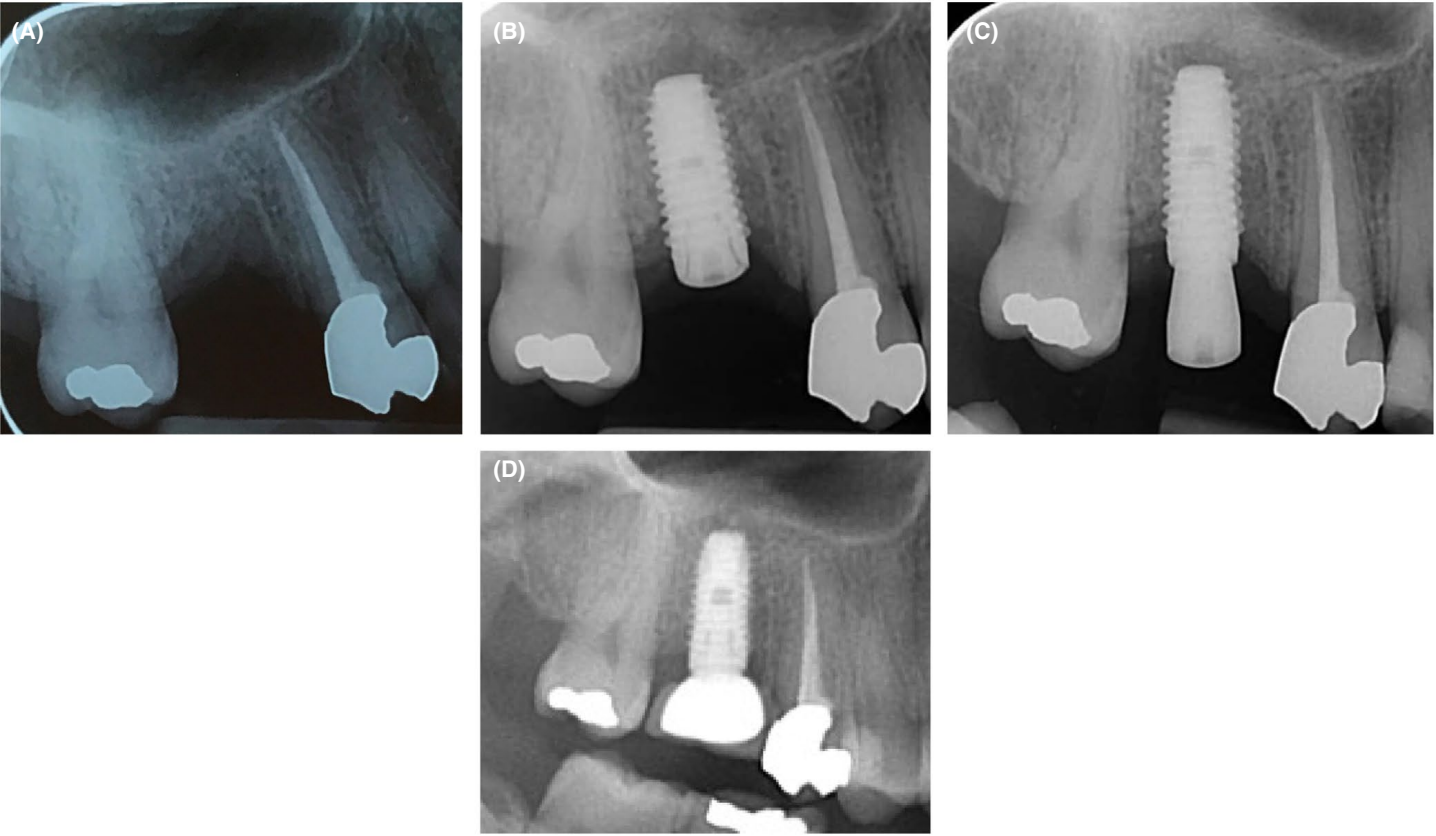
Intraoral radiographs of dental implant placement: A, baseline; B, on the day of implant insertion; C, after 6 mo, and D, last follow‐up after 20 mo
